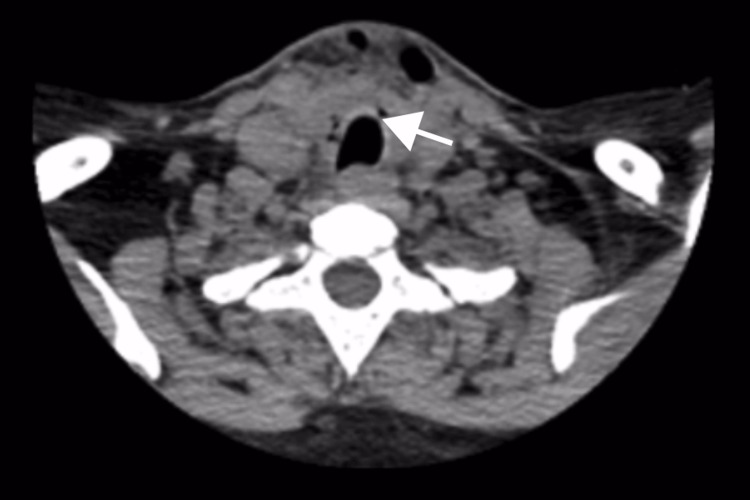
Axial noncontrast-enhanced computed tomography (NCCT) of the cervical spine showing an anterolateral tracheal defect. Axial view demonstrating a 1.5 mm defect (white arrow) in the anterolateral tracheal wall at the C7/T1 level.
